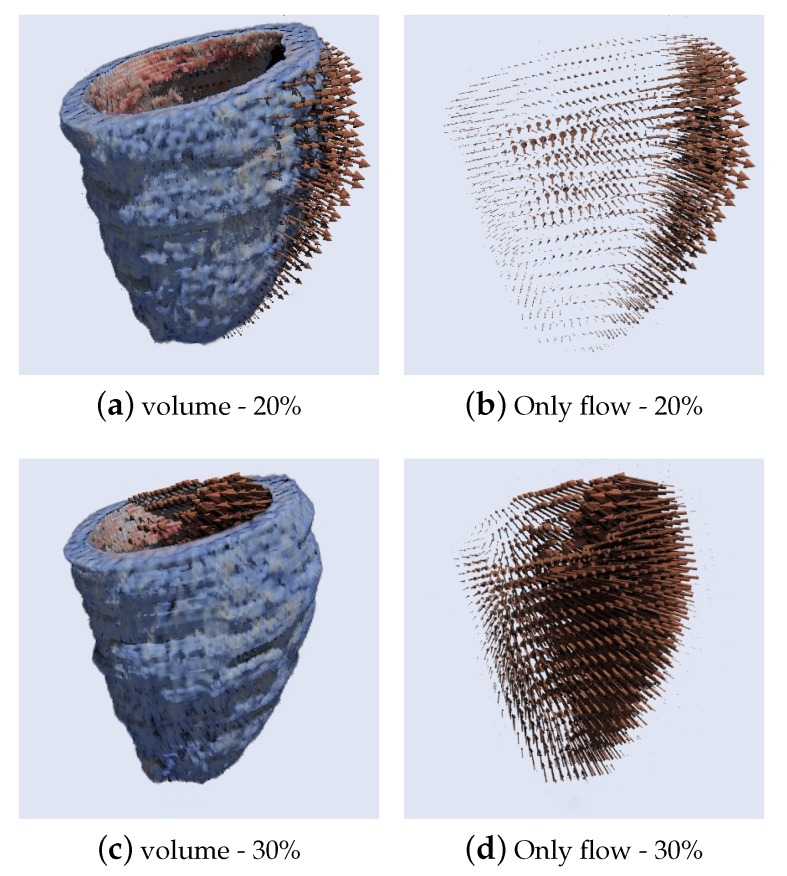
Results of 3D Optical Flow of a segmented cardiac CT volume (left ventricle) computed at phases 20–30% (contraction movement).
